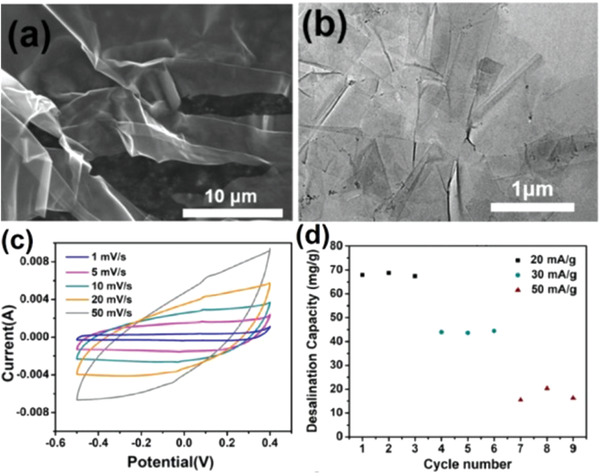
a) SEM images of Ti_3_C_2_T*_x_* after ultrasonic delamination, b) TEM images of Ti_3_C_2_T*_x_*, c) CV curves at different scan rates, d) desalination capacity at different current densities. Reproduced with permission.^[^
